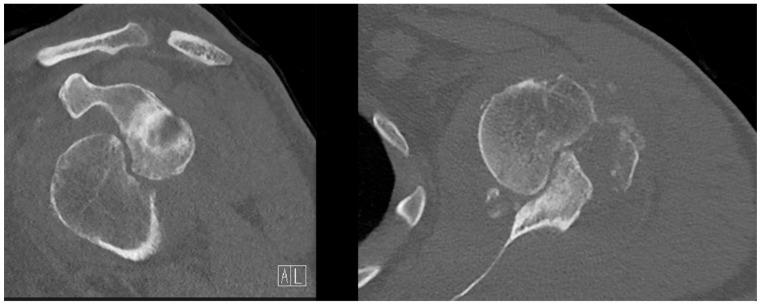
Preoperative CT scan. Transversal (right) and coronal (left) frames of the affected shoulder showing the large Hill-Sachs deformity. On the coronal image, the Bankart lesion on the anterior glenoid rim is clearly visible.
